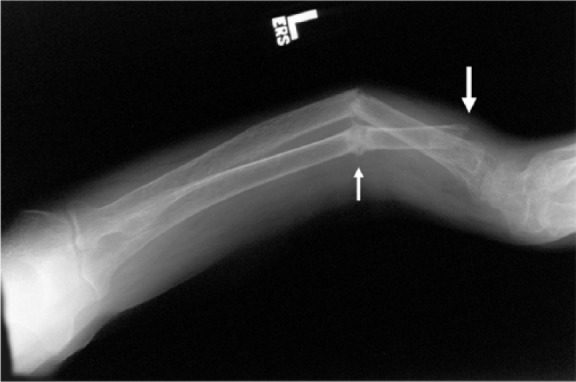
A radiograph of the left lateral forearm also shows marked bony demineralization. There are fractures at various stages of healing, as evidenced by callous formation at an old fracture (small arrow) and by sharp margins at an acute fracture (large arrow).
